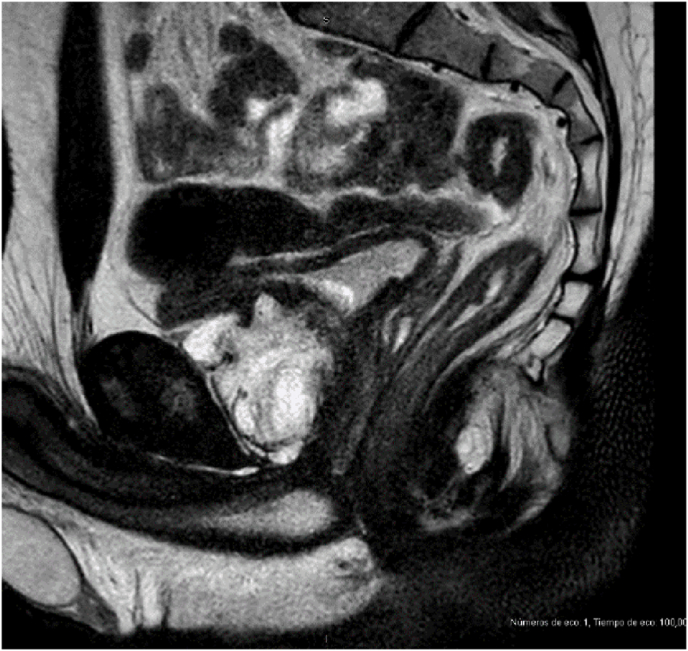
Prostate mass showed in MRI.
